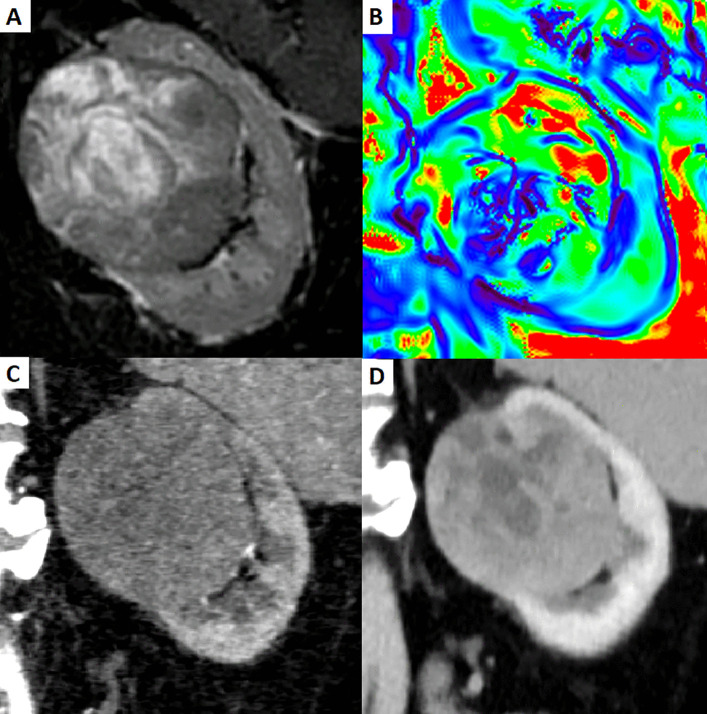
Example of corresponding slices in MRI and CT scans. Unclassified eosinophilic renal cell carcinoma (NOS), ISUP grade 3, measuring 7.9 cm in maximum diameter in the left kidney. The tumor is shown on (A) T2-weighted fat-suppressed MRI, (B) color-coded stiffness map obtained by MR elastography (shear wave velocity), (C) CT scan acquired during the cortical phase at 40 s, and (D) CT scan acquired during the nephrographic phase at 90 s. Multimodal imaging demonstrates tumor heterogeneity and supports the development of a biomimetic model based on these data.
